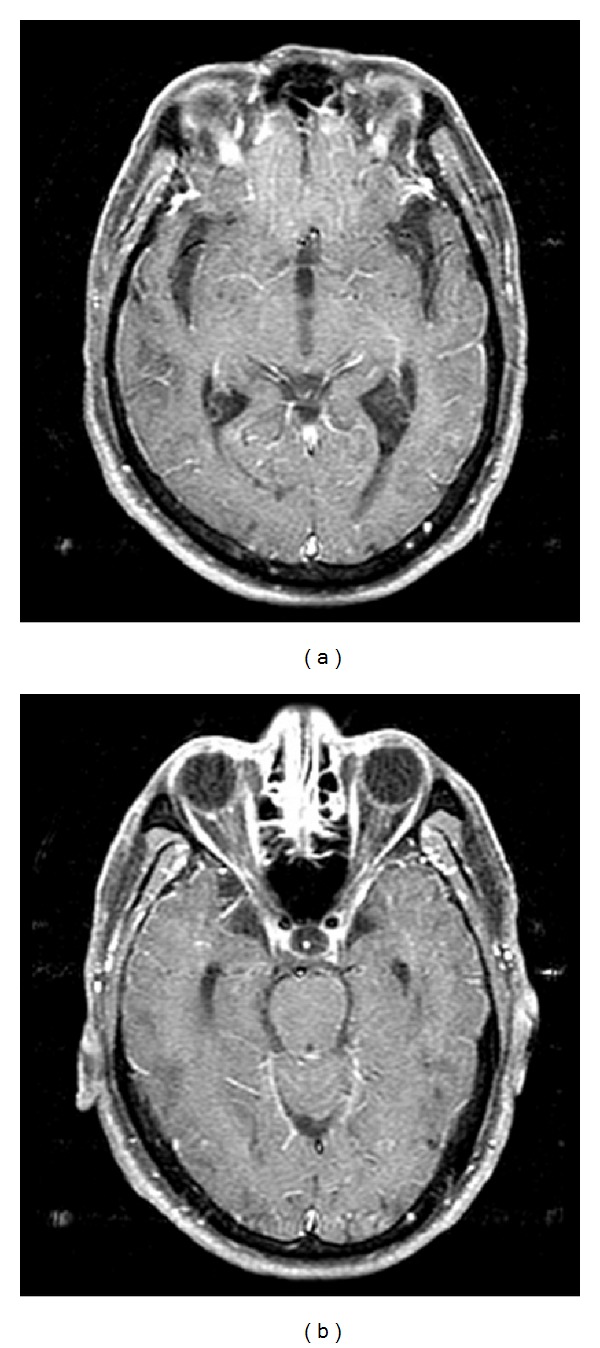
Brain magnetic resonance imaging with contrast showed mild diffuse ventriculomegaly and diffuse leptomeningeal enhancement, especially in posterior fossa area.
